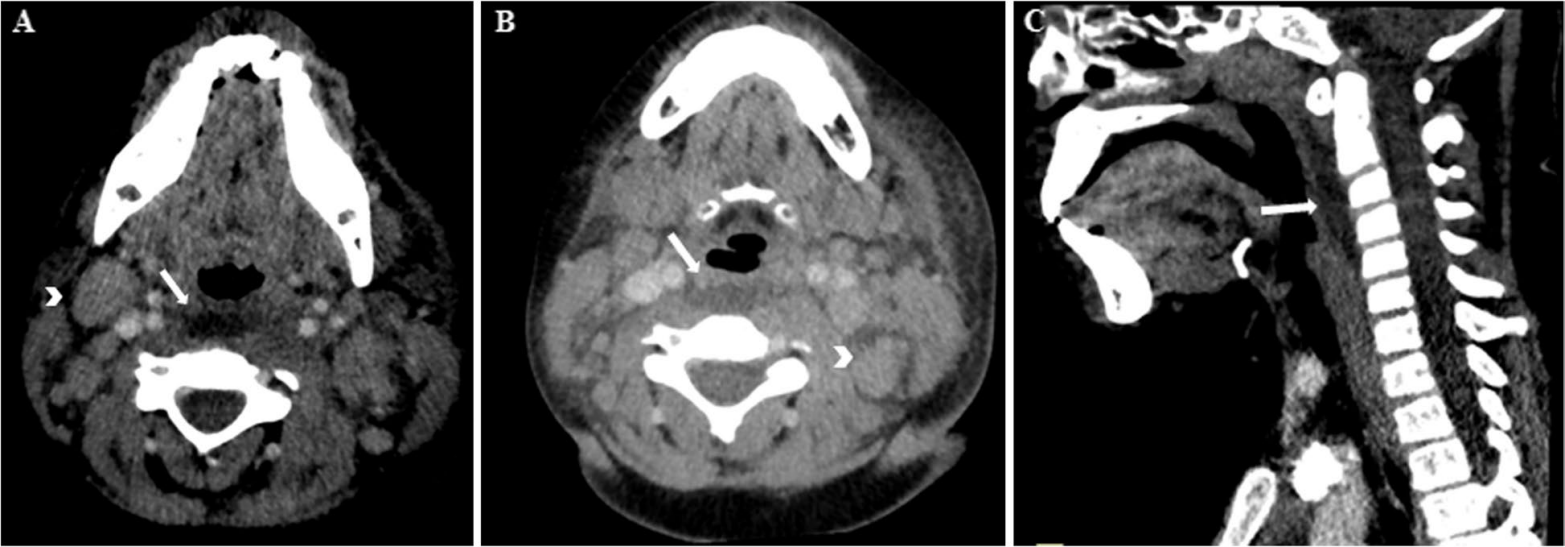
A and B Axial enhanced CT images demonstrate collection (arrows, A and B) filling from side to side in retropharyngeal space, with bilateral lymphadenopathy (arrowheads A and B). C Sagittal enhanced reformatted CT image shows fluid collection (arrow) expanding in retropharyngeal space
